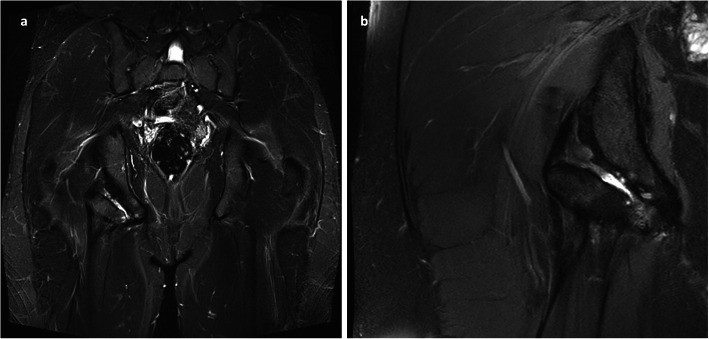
Preoperative MRI showed right anatomic footprint of hamstring tendons displaced from the ischial tuberosity (a, b)
